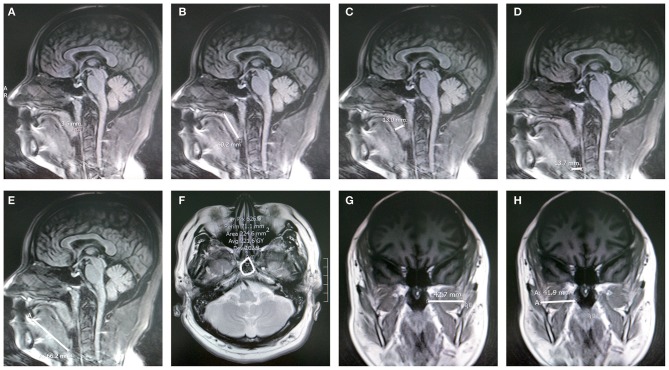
MRI measurements. (A) Retropalatal distance on a sagittal T1-weighted image. (B) Palatal length on a sagittal T1-weighted image. (C) Palatal thickness on a sagittal T1-weighted image. (D) Retroglossal space on a sagittal T1-weighted image. (E) Tongue length on a sagittal T1-weighted image. (F) Cross-sectional area of the retropharyngeal region on an axial T2-weighted image. (G,H) Lateral pharyngeal wall thickness on a coronal T1-weighted image.
